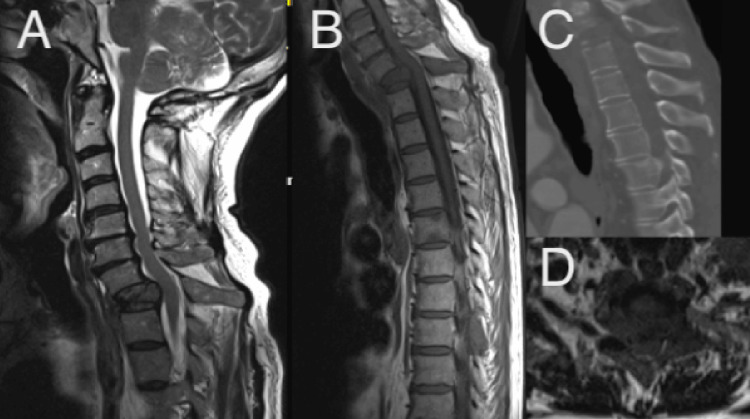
Metastatic disease at T1 with concomitant instability, treated with anterior corpectomy followed by Type 3 MICEPS. (A) MRI T2 sagittal plane; (B) MRI T1 sagittal plane; (C) CT sagittal plane; (D) MRI T2 axial view at the index level. MICEPS, minimally invasive cervical pedicle screw; MRI, magnetic resonance imaging
